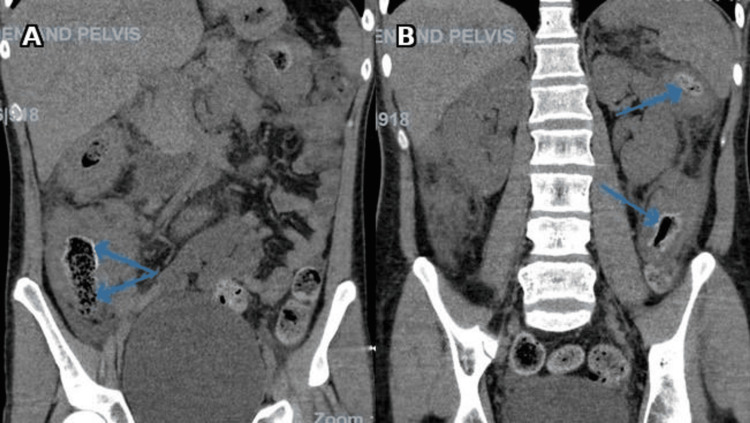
Contrast-enhanced CT of the abdomen and pelvis Coronal images show worsening luminal narrowing of the ascending colon with associated edema at the ileocecal junction (blue arrows) (A), and worsening luminal narrowing at the splenic flexure and descending colon (blue arrows) (B).
